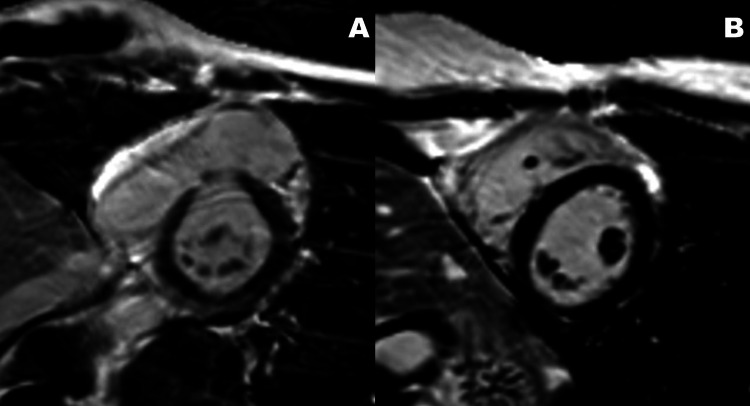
Cardiac MRI with gadolinium A. Short axis at the level of the mitral valve. B. Short axis at the level of papillary muscles.
